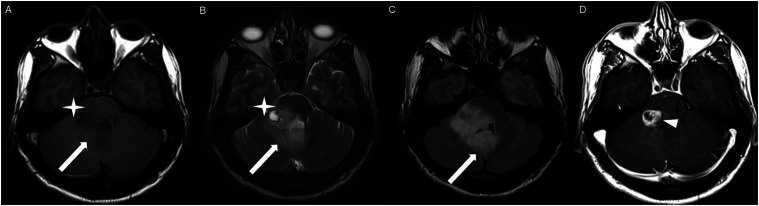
Axial T1-weighted image (A), axial T2-weighted image (B), axial FLAIR (C) and axial post-contrast T1 image (D), show a T1 hypointense, T2/FLAIR hyperintense mass (A-C, thick white arrow) involving the right middle cerebellar peduncle, right side of the pons and midbrain and cerebellar vermis, with mixed non-enhancing and nodular-enhancing components (D, arrowhead). Focal cystic changes are also seen on T2-weighted image (B, star).
